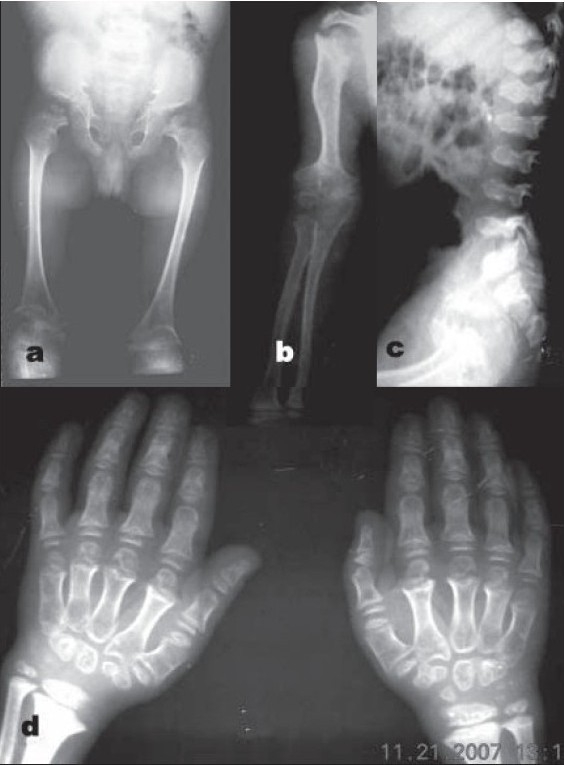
8 year old (elder) sibling. (a) Radiograph (anteroposterior view) of pelvis reveals squared ilium, narrow sacrosciatic notches, dysplastic acetabuli, and a characteristic medial beak at femoral neck. (b) Radiograph (anteroposterior view) of upper limb showing markedly flared and irregular metaphysis with deformed, irregular, and fragmented epiphyses. (c) Lateral radiograph of Lumbosacral spine showing platyspondyly with central beaking. (d) Radiograph (anteroposterior view) of hand shows underdeveloped carpals with short and broad metacarpals and phalanges
